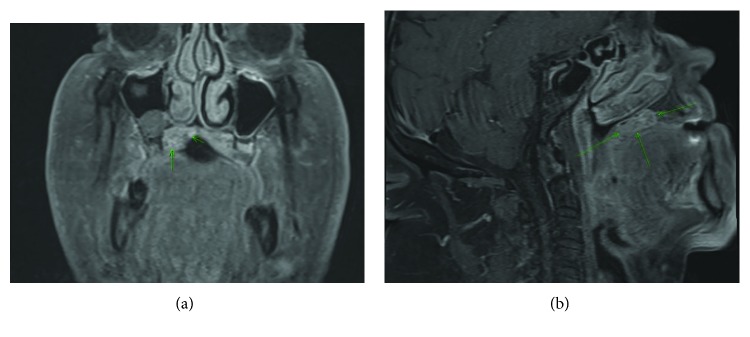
(a) MRI coronal T1image with MASC tumor. (b) MRI sagittal T1 image with MASC tumor.
